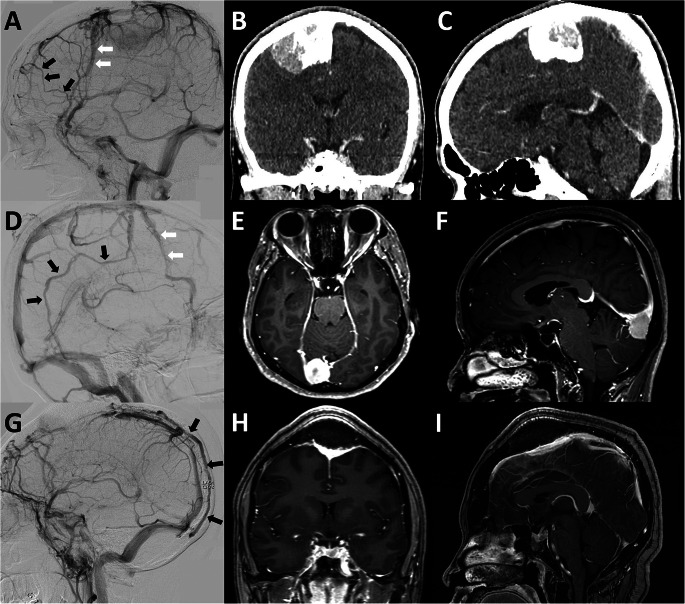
DV route patterns. DSA and brain imaging studies in case no.3 (A-C), 4 (D-F), and 5 (G-I). (A) PFO route of DV (black arrows), PFP route of DV (white arrows) on DSA; (B, C) contrast-enhanced cranial CT in coronal and sagittal views, pathological diagnosis was meningioma; (D) OP route of DV (black arrows), PFP route of DV (white arrows) on DSA; (E, F) contrast-enhanced cranial MRI in axial and sagittal views, pathological diagnosis was meningioma; (G) OC route of DV (black arrows) on DSA; and (H, I) contrast-enhanced cranial MRI in coronal and sagittal views, pathological diagnosis was meningioma. DSA, digital subtraction angiography; DV, diploic vein; OC, occipitocervical; OP, occipitoparietal; PFO, pterional part of fronto-orbital; PFP, pteriofrontoparietal
